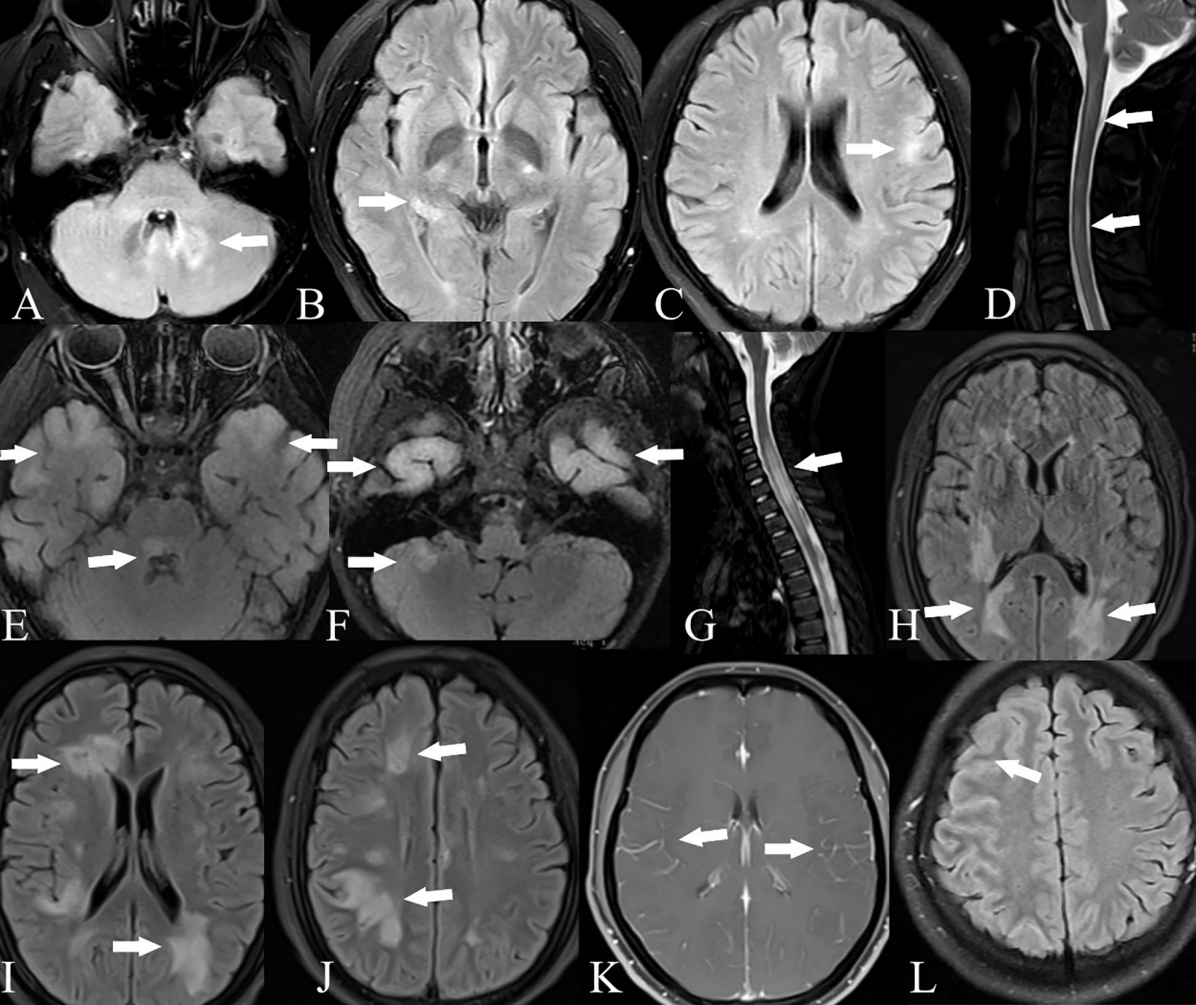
Brain and spinal cord MRI of GFAP-MOG overlapping syndrome. Patient 11: T2-FLAIR imaging showed lesions in the cerebellum (A), thalamus (B), and cortex (C); T2-weighted imaging showed lesions in the spinal cord (D). Patient 13: T2-FLAIR imaging showed lesions in the bilateral cortex, pons, and cerebellum (E, F); T2-weighted imaging showed lesions in the spinal cord (G). Patient 14: T2-FLAIR imaging showed lesions in the bilateral cortex, periventricular region, and basal ganglia (H–J). Patient 15: Contrast-enhanced T1-weighted imaging showed leptomeningeal enhancement (K). Patient 16: T2-FLAIR imaging showed lesions in the right frontal and parietal cortex (L).
